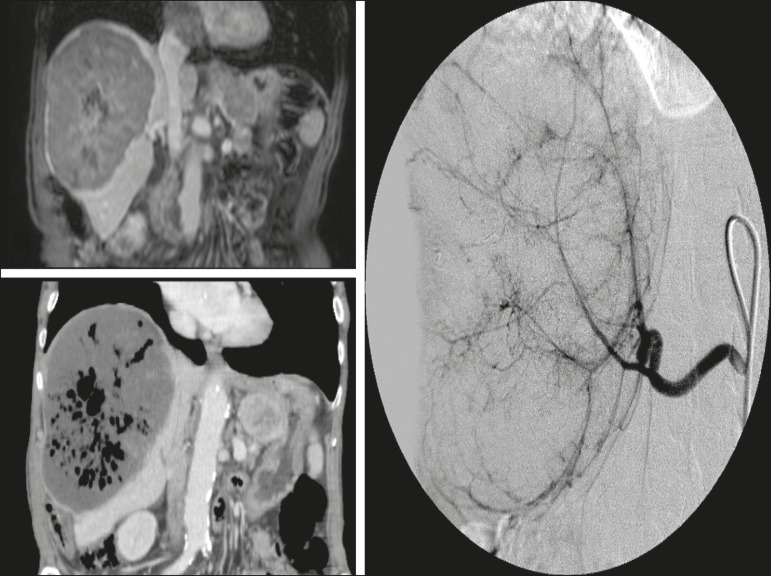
An 82-year-old male patient with an HCC, of the fibrolamellar subtype, measuring 18 cm and located in the right postero-inferior and postero-superior lateral segments (segments VI and VII, respectively) of the liver, as seen on an unenhanced magnetic resonance imaging scan. DEB-TACE of the liver tumour was performed with two vials of HepaSphere 150-200 µm (Merit Medical Systems, South Jordan, UT, USA) loaded with doxorubicin 75 mg. The patient evolved to an excellent general health status, with no signs of infection. A follow-up computed tomography scan, acquired one month later, showing 100% necrosis with intratumoural gas related to the tumour necrosis.
